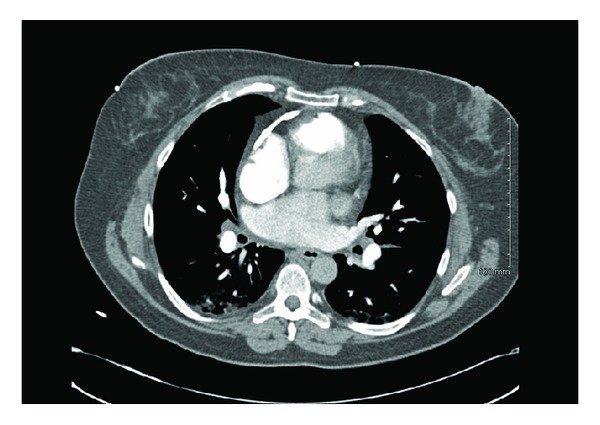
CT scan of chest showing pericardial calcifications.
